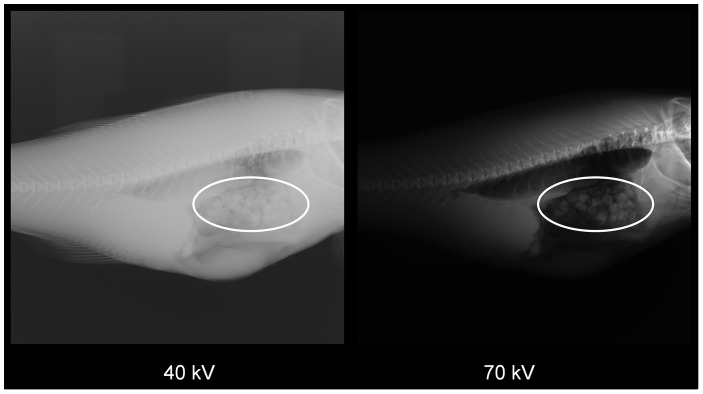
Dual energy X-ray images of Atlantic cod: a) X-ray image at 40 kV, b) X-ray image at 70 kV.
